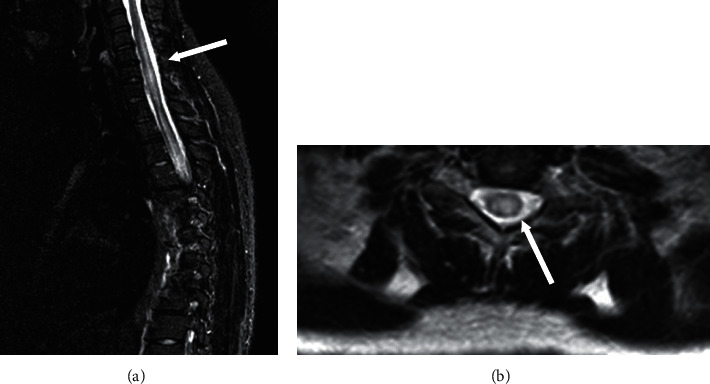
Initial MRI of the cervical and thoracic spine. (a) Sagittal short tau inversion recovery (STIR) image shows increased signal intensity of the spinal cord beginning at C4 with caudal extension into the thoracic spine. (b) T2-weighted axial image at the C4 level demonstrates increased central signal intensity of the spinal cord.
